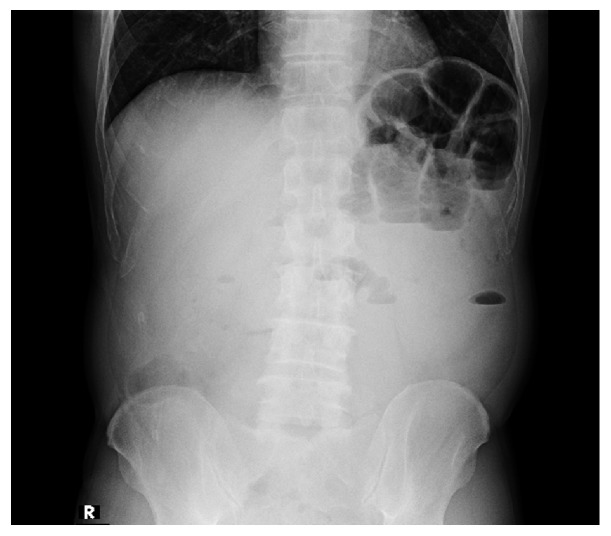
Abdominal radiography performed in upright position revealed distended loops of small bowel containing gas and fluid in the left upper abdomen.
